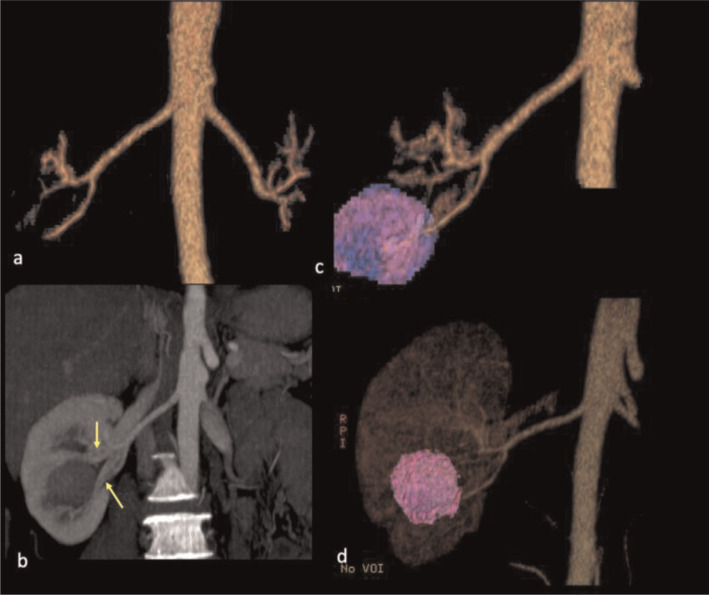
A 46-year-old female (case 4) had a 2.2 cm right renal tumor. (a) The 3D CT arteriography of the aorta and bilateral renal arteriogram was reconstructed first. (b) The maximum intensity projection (MIP) showed the relationship of the poorly enhanced renal tumor and adjacent renal venous branches (arrows). (c) The 3D CT angiography was merged with the 3D-surface rendered tumor to show that the blood supply of the renal tumor was from the inferior segmental artery. (d) The 3D CT angiography was merged with the 3D surface-rendered tumor and renal shadow to demonstrate the location of the tumor in the right kidney.
